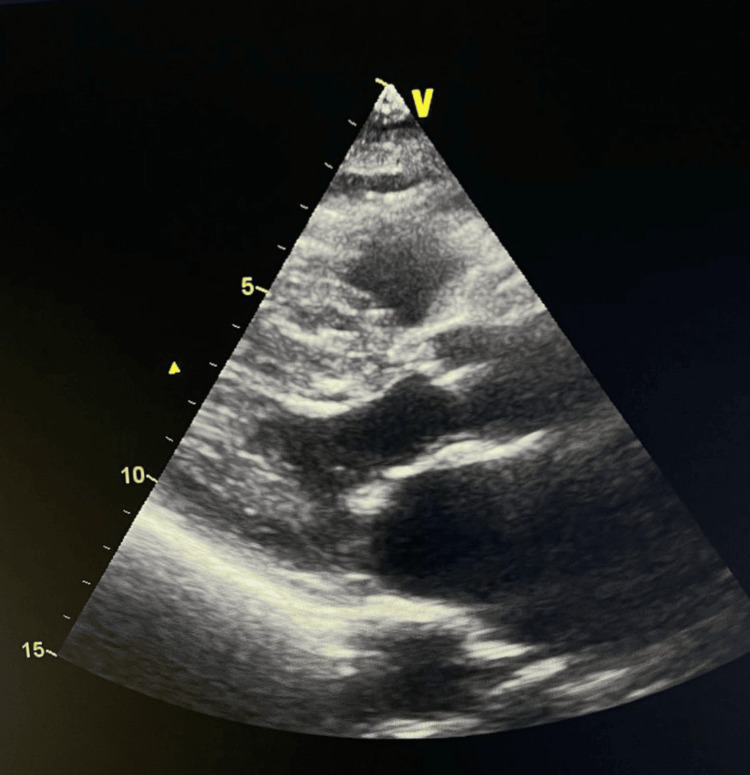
Parasternal long-axis view demonstrating ventricular hypertrophy. Parasternal long-axis (PLAX) transthoracic echocardiographic image showing marked left ventricular hypertrophy involving the mid-ventricular segments. Echogenicity is suboptimal, limiting precise visualization of the apical region, but the degree of hypertrophy is clearly appreciable. This pattern is suggestive of obstructive hypertrophic cardiomyopathy phenotype.
